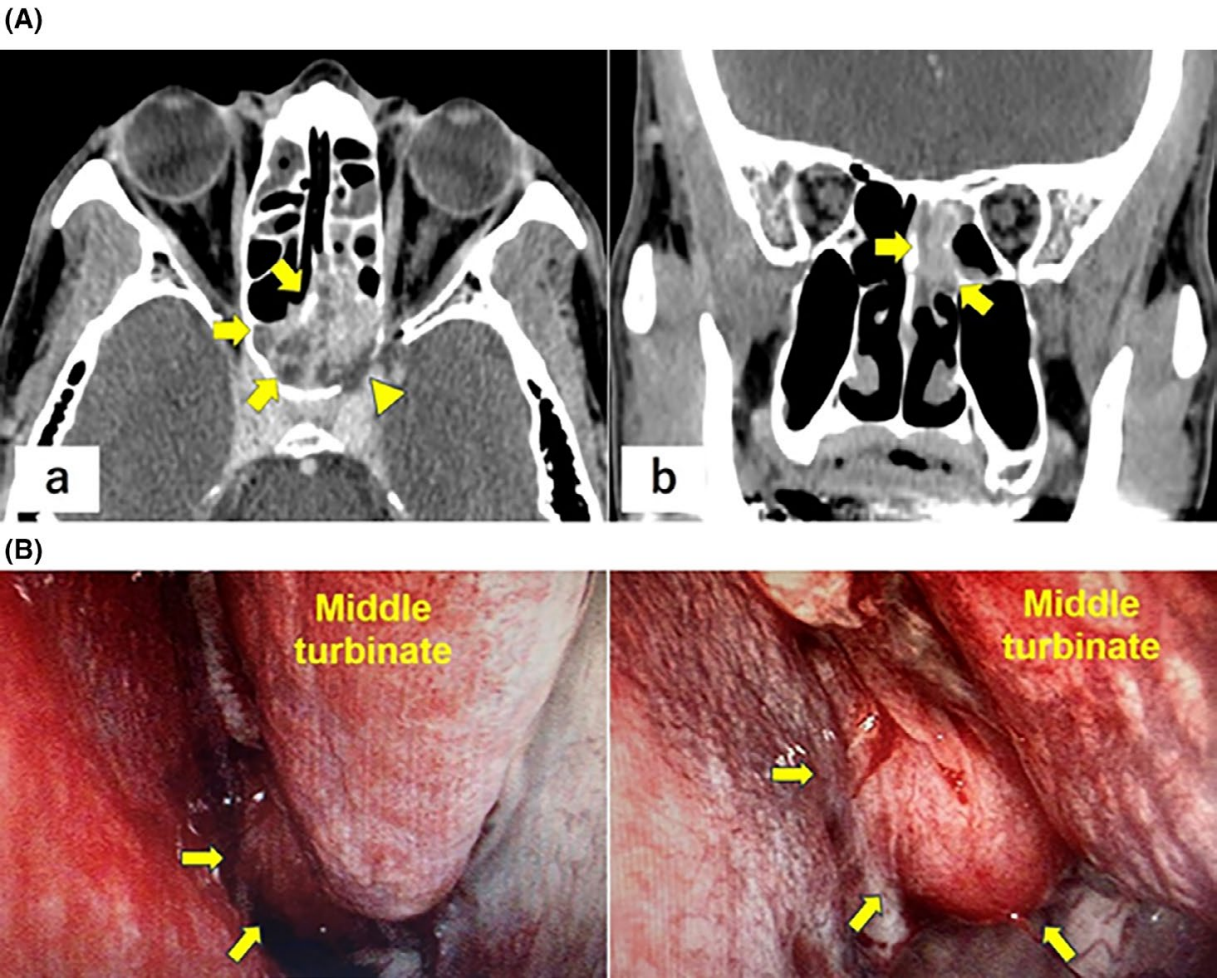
Contrast‐enhanced head computed tomography images at presentation demonstrating an approximately 4‐cm hyper vascular mass centered in the sphenoid sinus with osseous destruction involving the sellar floor and clivus. (A) Axial image. (B) Coronal image. Arrows: Tumors; arrowheads: Osseous destruction. (B) Nasal endoscopic view demonstrating a tumor located posterior to the left middle turbinate. Arrows: tumors. [Correction added on 20 March 2026, after first online publication: Figure 1 and its caption have been corrected to show previously missing bottom panels.]
